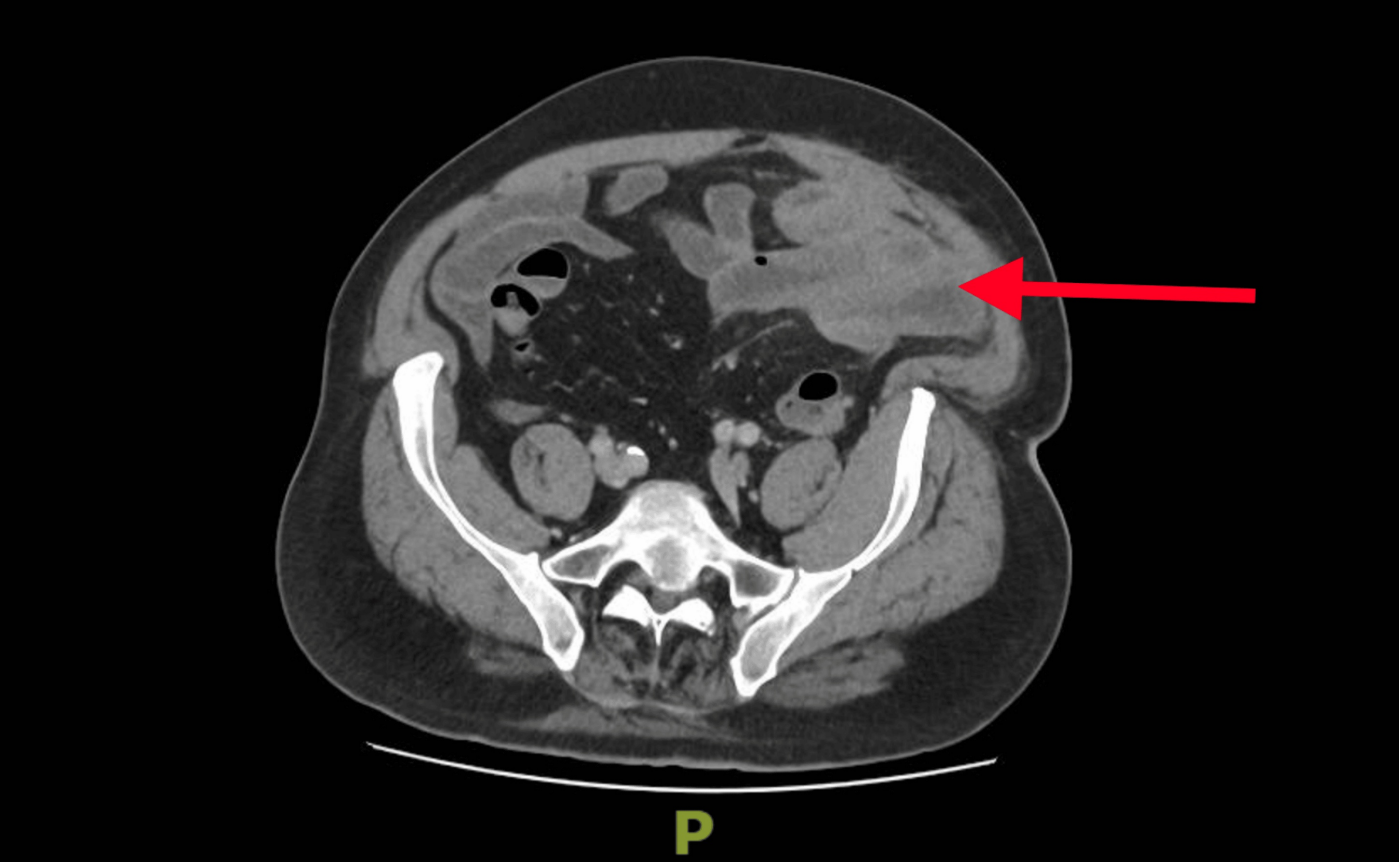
Axial view (superior slice) of contrast-enhanced CT of the pelvis The red arrow highlights adjacent small bowel loops showing mural hyperenhancement and mild edema, representing reactive enteritis secondary to adjacent sigmoid diverticulitis.
